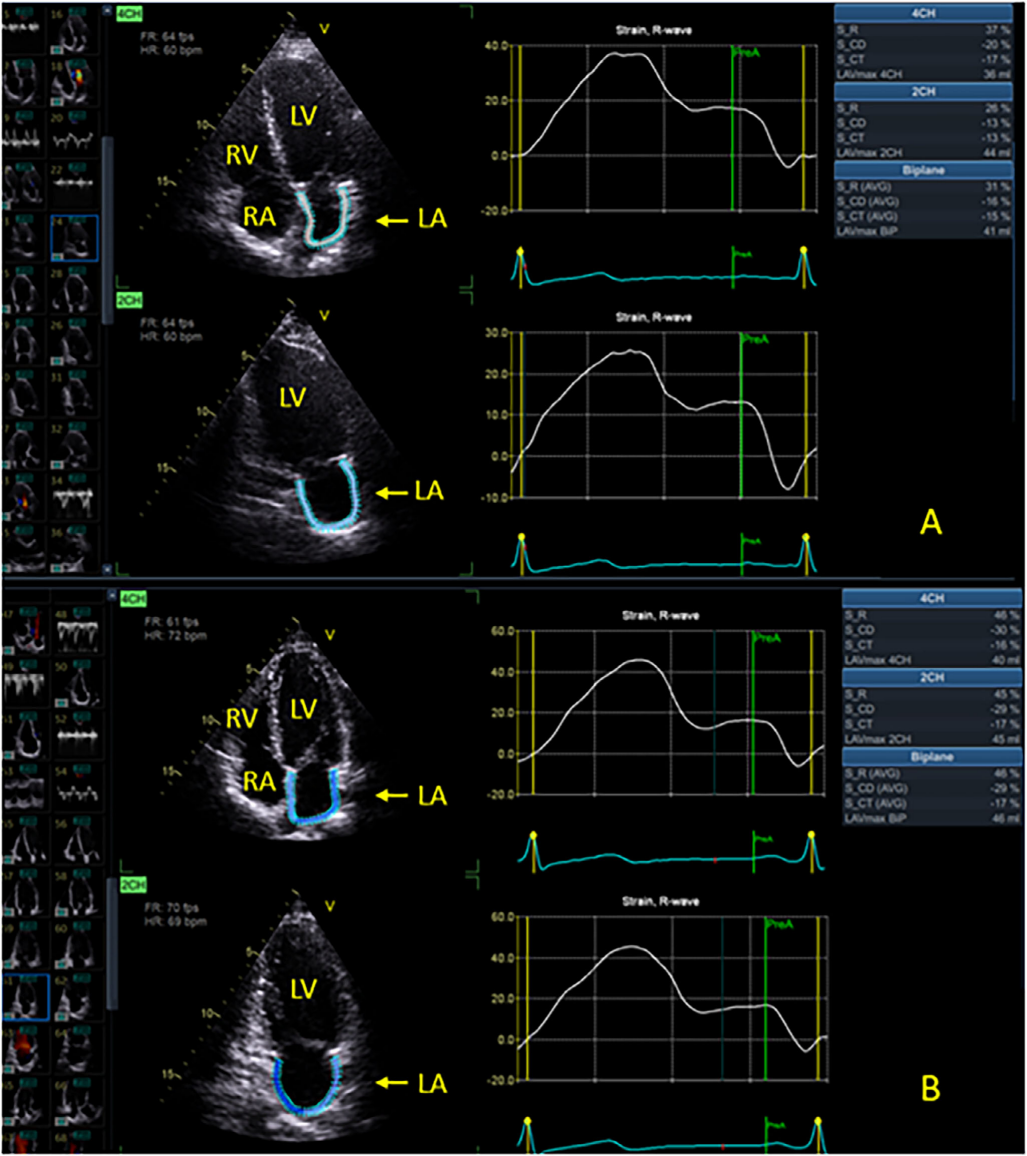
Normal General Electric EchoPAC left atrial strain before and after exercise (*normal SE*). (A) Pre‐exercise LAS in the A4C and A2C. (B) Post‐exercise LAS. A4C, apical four chamber; A2C, apical two chamber; LAS, left atrial strain; SE, stress echocardiogram.
